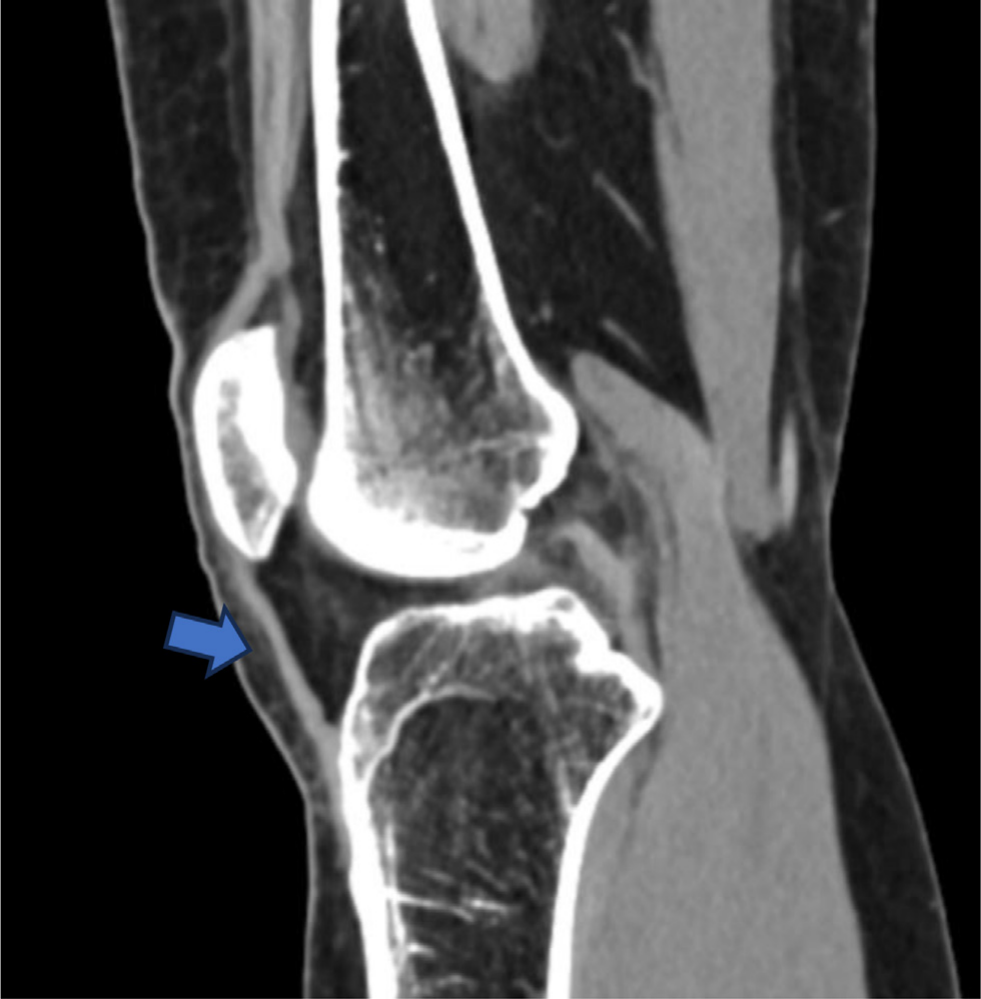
Sagittal CT image of the right knee (bone window) Sagittal computed tomography (CT) image showing a low-riding patella in relation to the femoral trochlea, consistent with patella baja. Blue arrow indicates the shortened patellar tendon.
